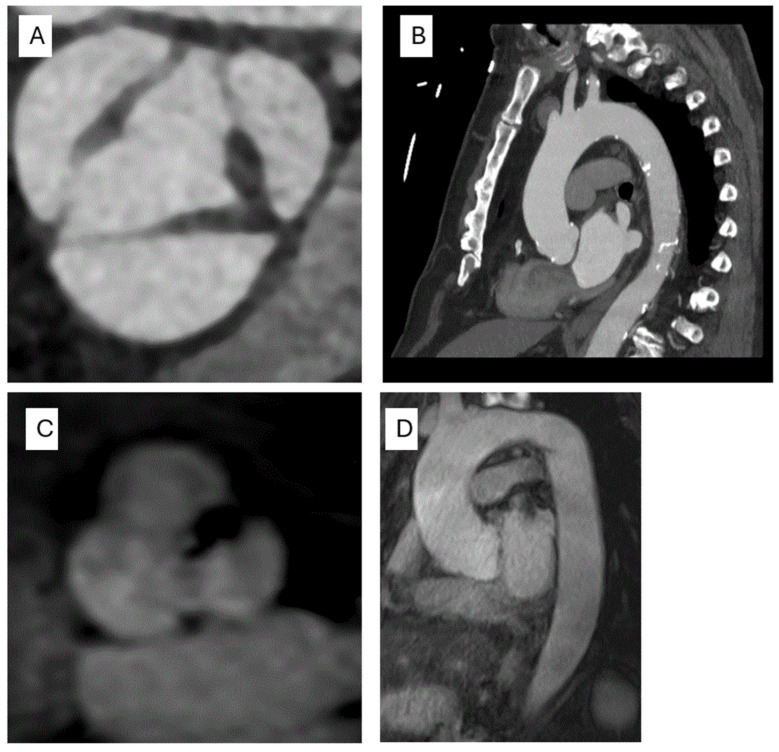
Evaluation of the aortic valve and thoracic aorta by CTA and CMR. (A) Short axis of the aortic valve reconstructed to mimic a short-axis view demonstrating BAV with right and left coronary cusps and a raphe at the commissure. (B) Visualization of the entire thoracic aorta using MPR. (C) Short axis of the aortic valve suggesting the fusion of the right and left coronary cusps. (D) CMR allows for the evaluation of the entire aorta and offers MPR. CMR: Cardiac Magnetic Resonance; BAV: Bicuspid Aortic Valve; MPR: Multi-planar Reconstruction; CTA: Computed Tomography Angiography.
